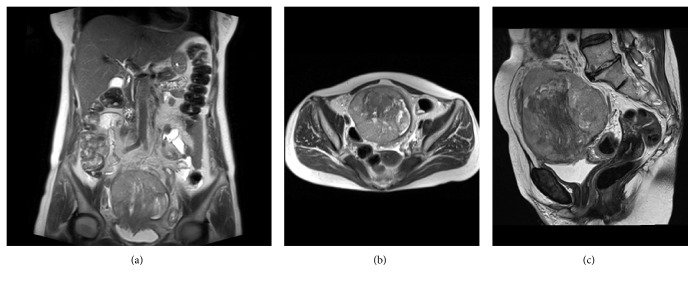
Abdominopelvic Magnetic Resonance Image (MRI) showing a 12 × 11 × 10 cm mass with necrotic center and heterogeneous signal on T2-weighted sequence. Coronal (a), axial (b), and sagittal (c) MRI images revealed the mass located at the umbilicus extending to the bladder dome, which is highly suggestive of urachal cancer.
